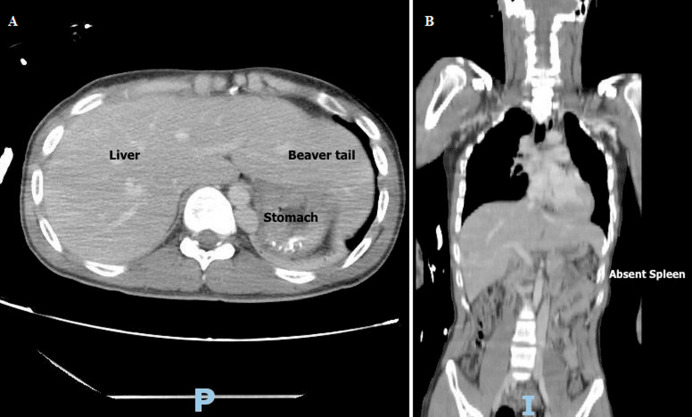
(A,B) axial and coronal views of the CT scan showing a beaver tail liver and asplenia
